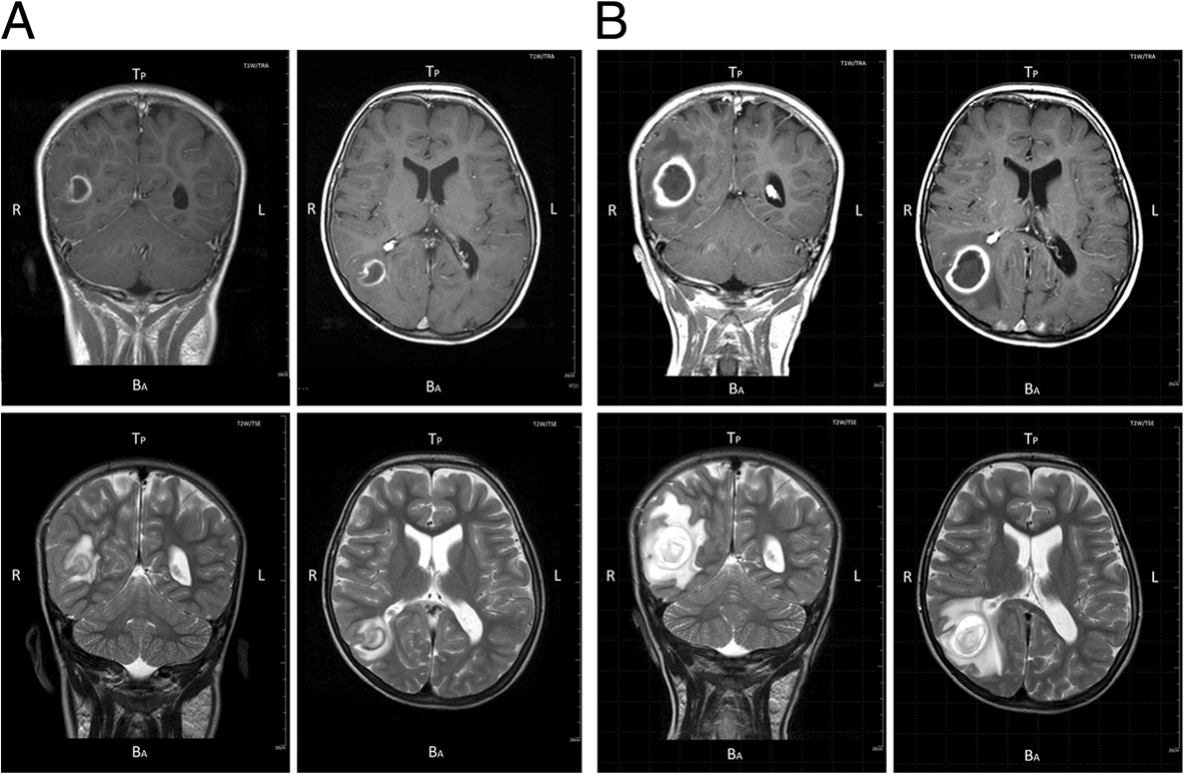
A magnetic resonance imaging of the brain demonstrating a ring-shaped abscess of 18 mm on August 8 (a) and that of 29 mm on August 22 (b)
